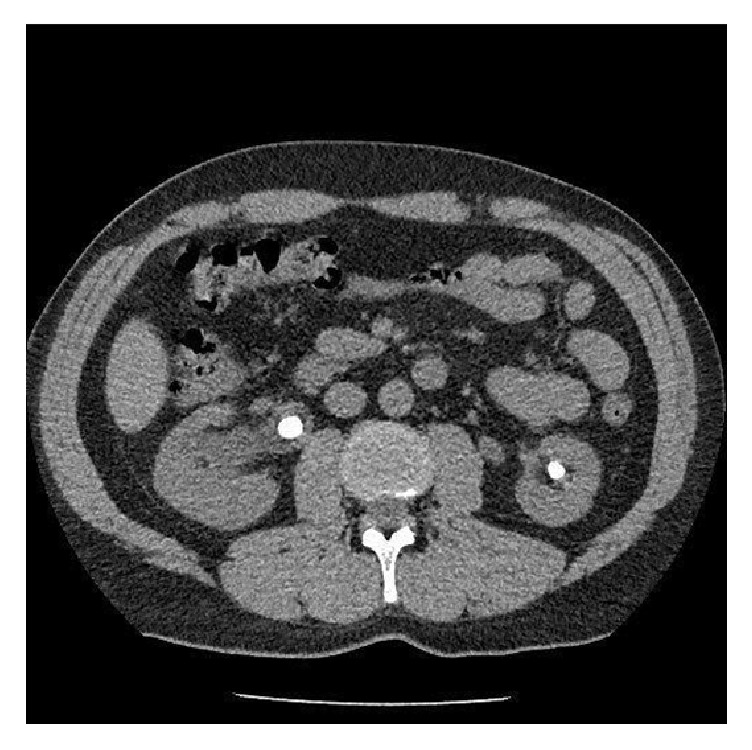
Preoperative CT scan. Bilateral nephrolithiasis.
